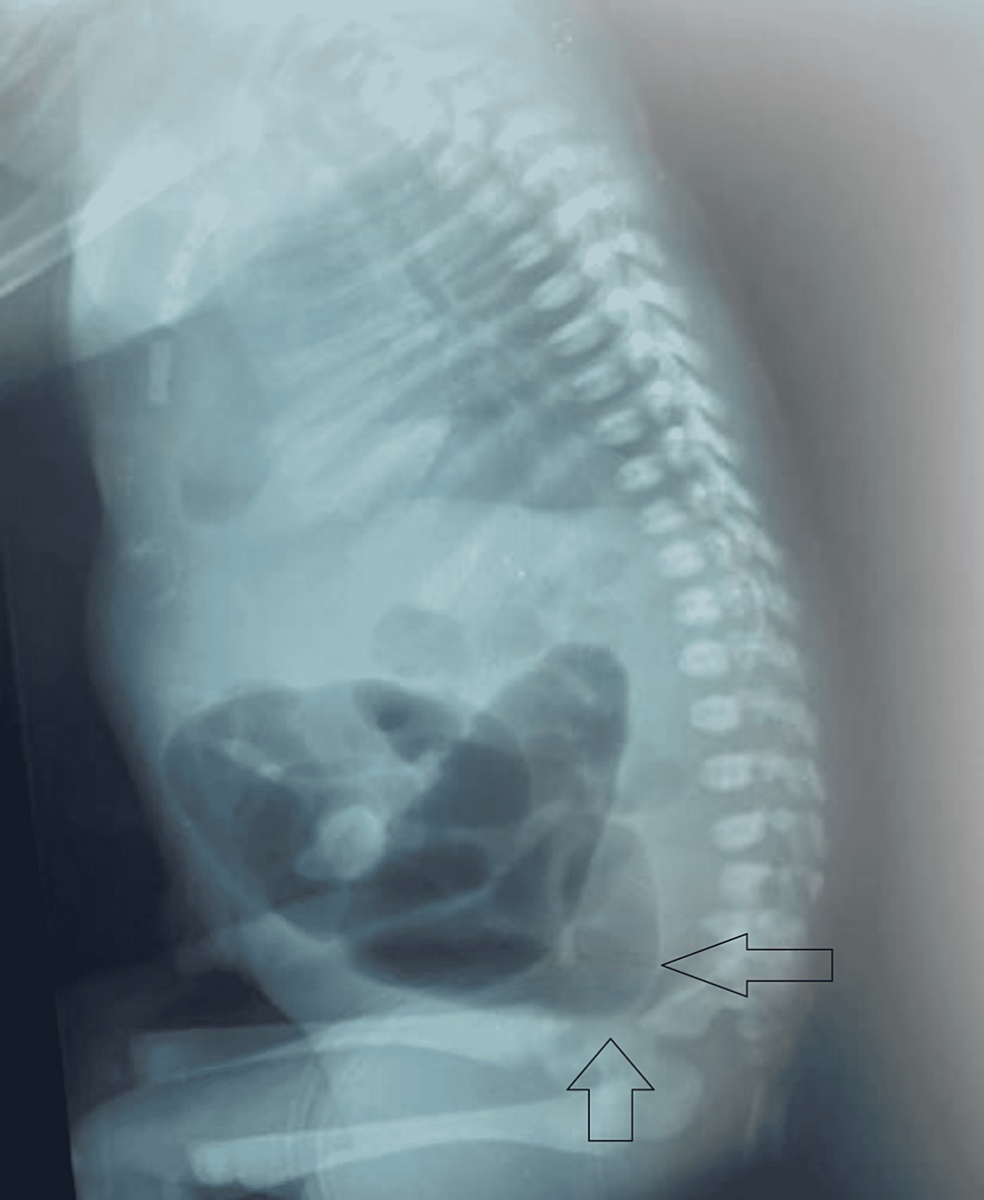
Abdominal X-ray; lateral decubitus view shows dilated gut loops with absence of air at the site of rectum near pelvis.
